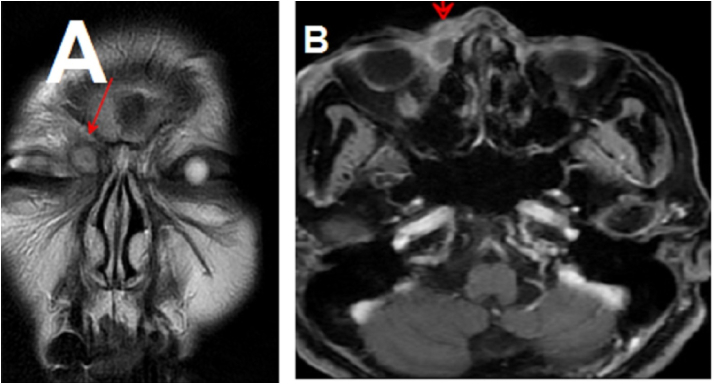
A&B: MRI orbit showing: Well defined cystic biloculated lesion seen implicating the medial inferior corner of the right orbit at the region of the lacrimal sac, it is seen extending into the right nasolacrimal duct.
